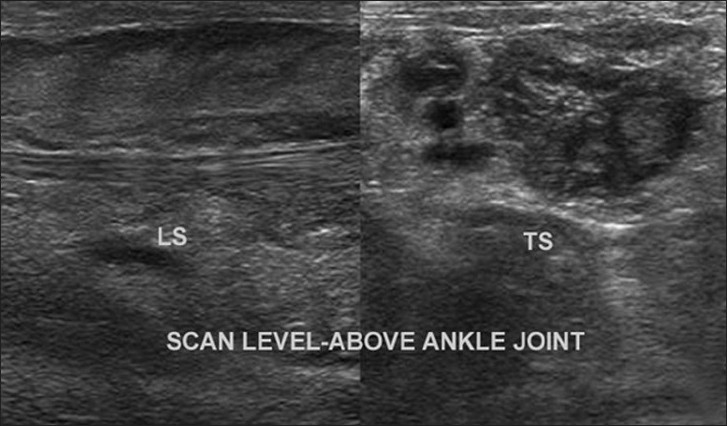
Gray scale ultrasonography of the right lower limb shows multilobulated tortuous entanglement of tumors, oriented along the long axis of the nerve on longitudinal section, and “target sign” on transverse scan, evident as echogenic center and hypoechoic periphery
